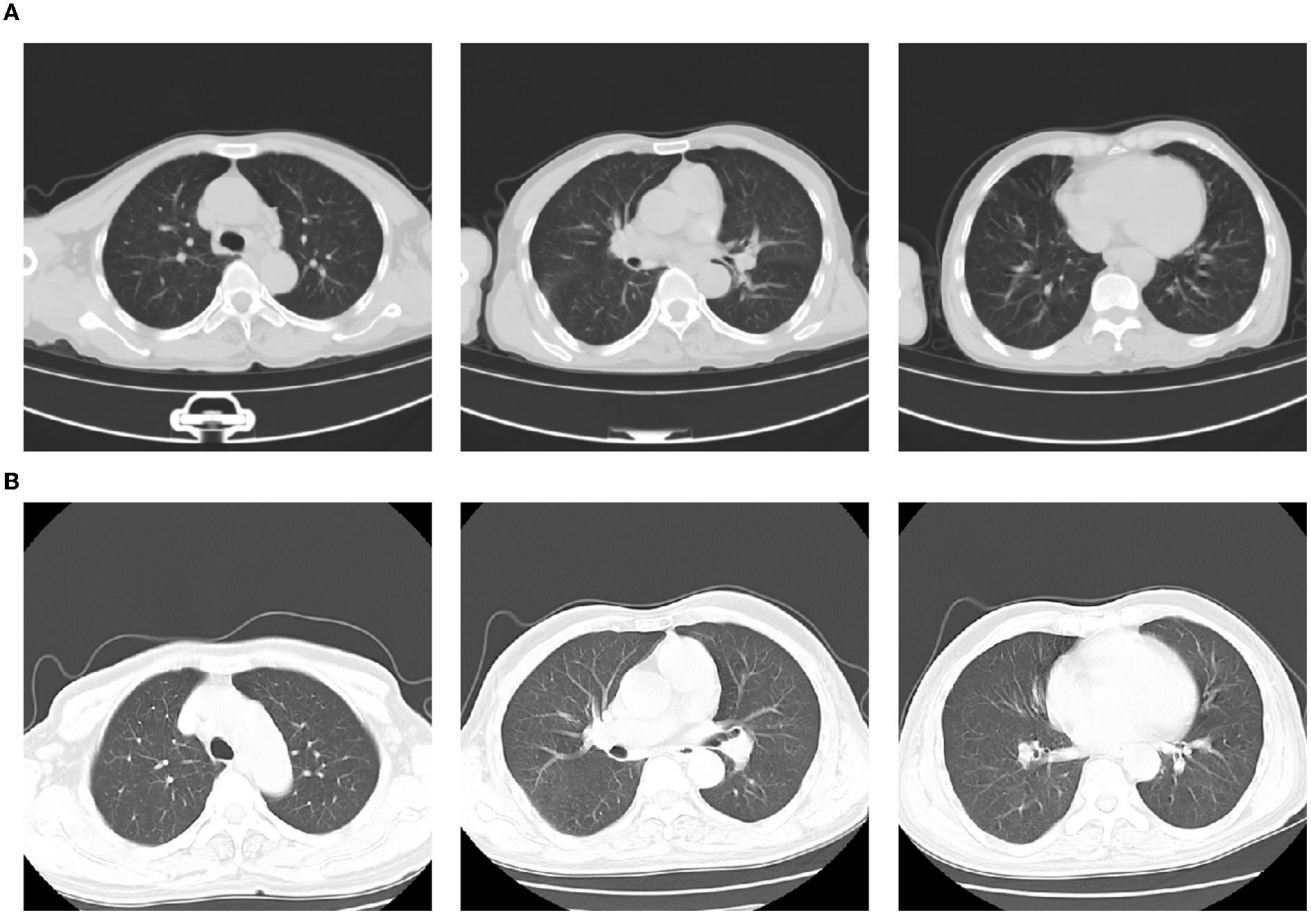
Computed tomography (CT) on admission showing chronic bronchitis with bilateral lung infection (A); CT presentation on day 14 of hospitalization (day 3 of meropenem administration): No obvious lesions in both lungs. However, multiple enlarged lymph nodes are seen in the mediastinum and bilateral axillae (B).
